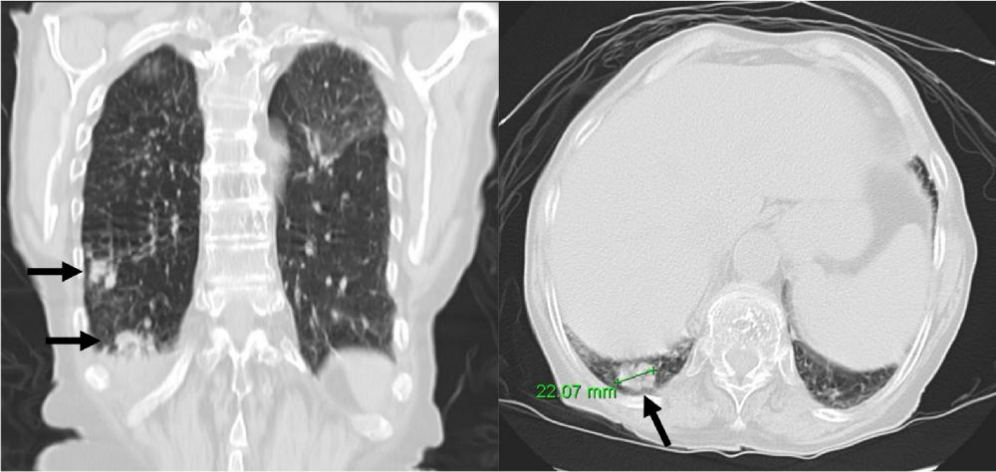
Nodular formations on thoracic computed tomography (arrows).
